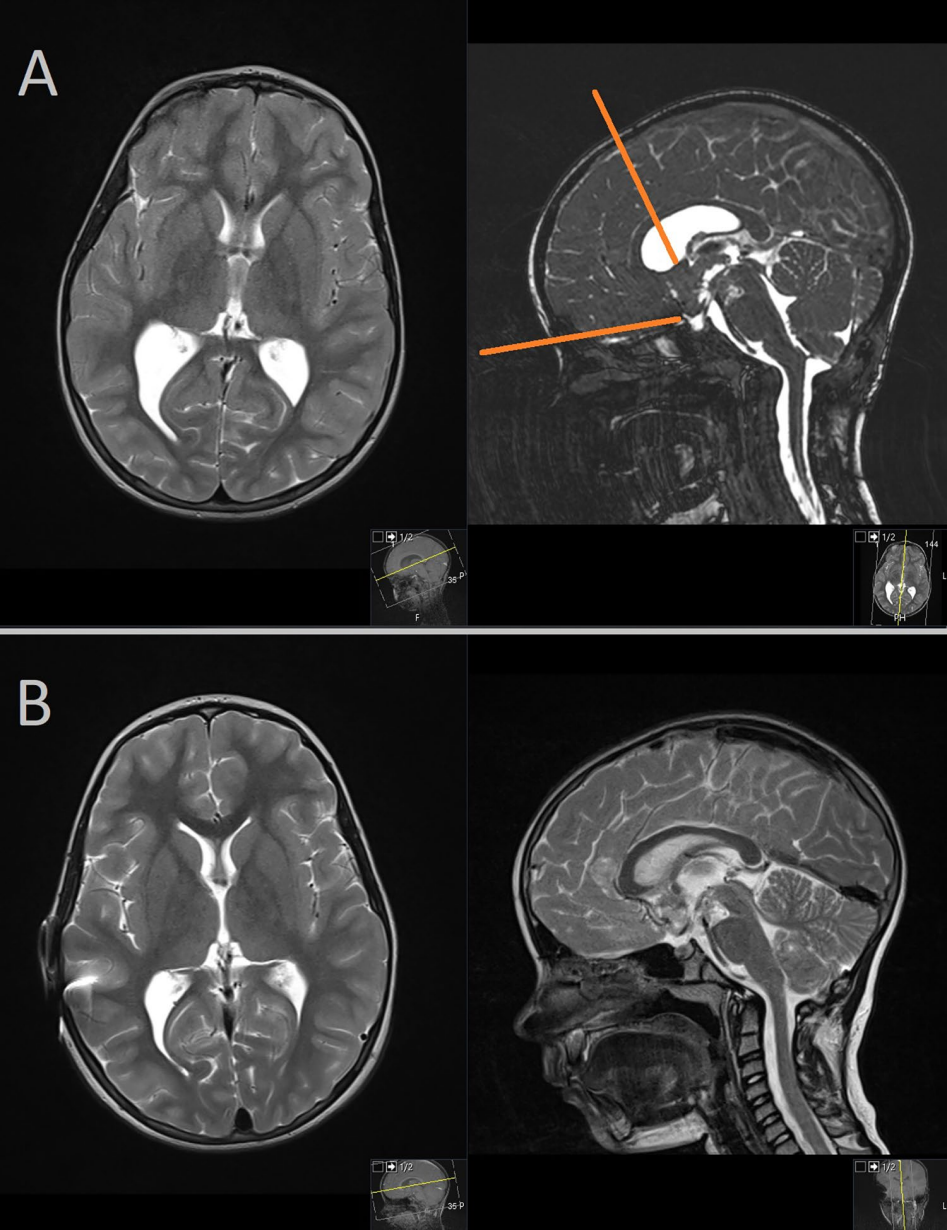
A MRI examination revealing a third ventricular tumor with frontobasal extension. B Radiological imaging demonstrating good tumor control without signs of progression
